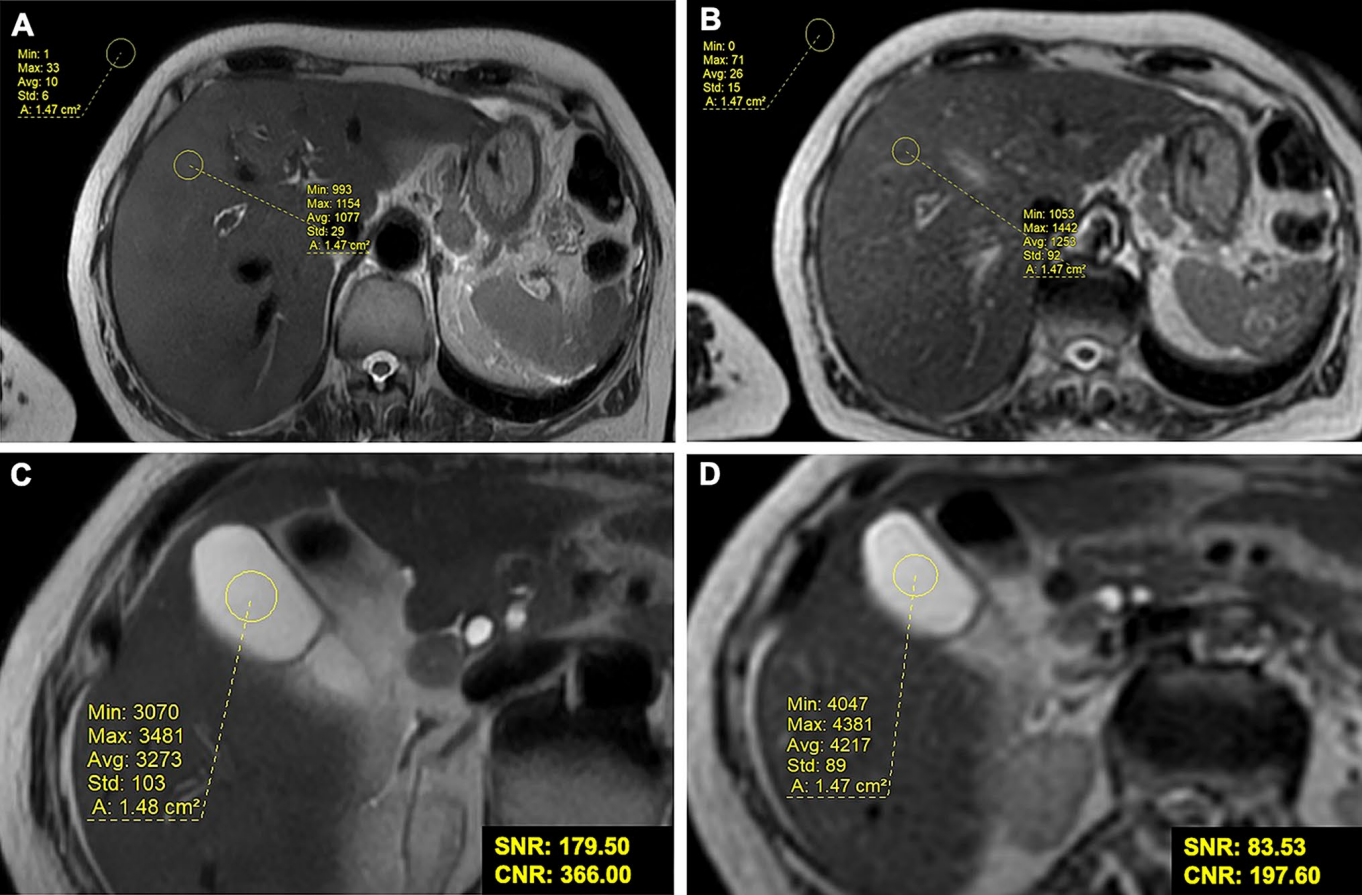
ROIs placement on MRI scan for quantitative analysis. Unenhanced axial SSFSE T2 images of a 52-years-old male with one of the three ROIs placed on the V hepatic segment liver, one ROI on the background and one in the gallbladder with ARDL a, c NAÏVE b, d showing significant differences in SNR and CNR between ARDL and NAÏVE images
